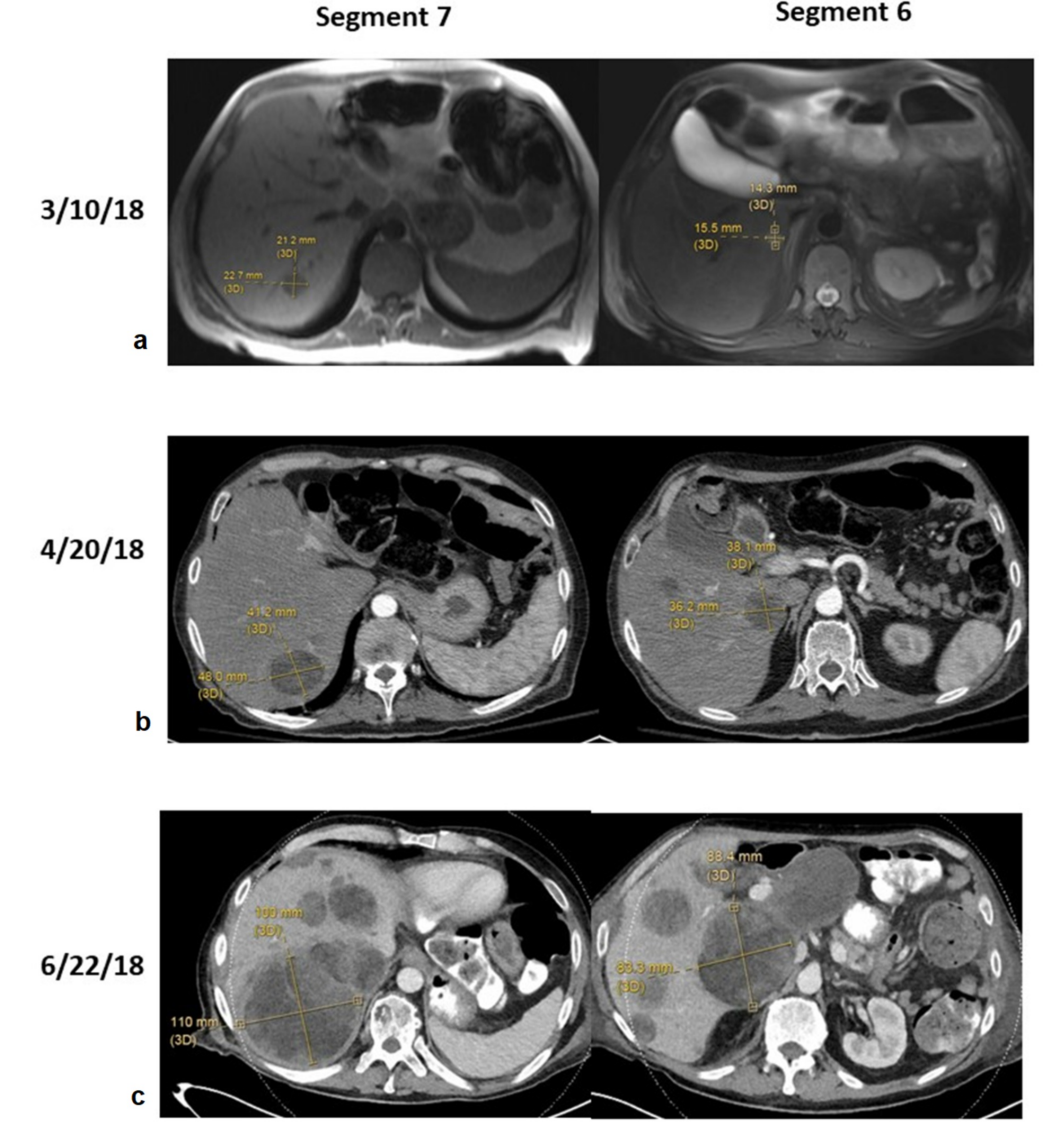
MRI and CT images of segment 6 and 7 at three different time points a) On March 10, 2018, segment 7 was 2.1 x 2.3 x 1.9 cm and segment 6 was 1.5 x 1.4 cm; b) on April 20, 2018, segment 7 was 4.8 x 4.1 cm and segment 6 was 3.8 x 3.6 cm; c) on June 28, 2018, segment 7 was 10.1 x 9.1 x 10 cm and segment 6 was 8.3 x 8.8 cm CT: computed tomography; MRI: magnetic resonance imaging
